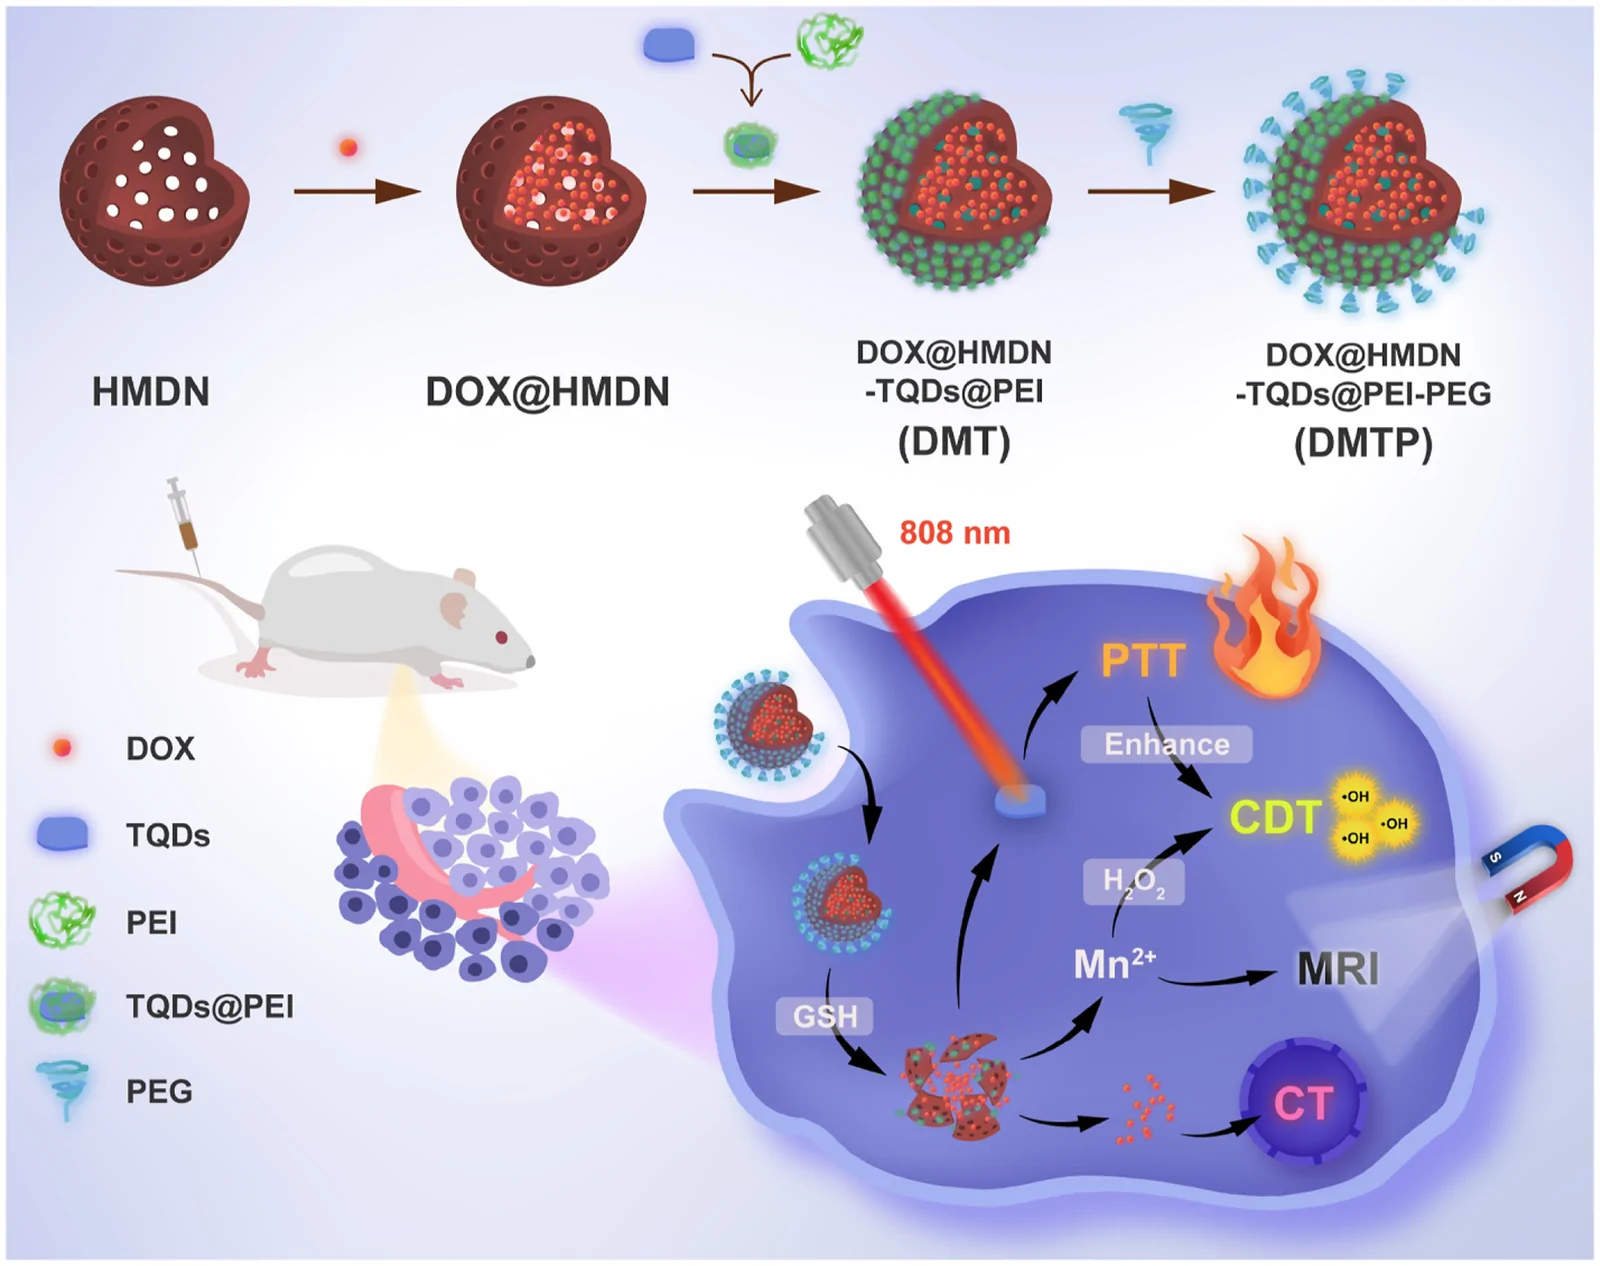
Synthesis of DOX@HMDN–TQDs@PEI–PEG (DMTP), CDT and MRI. This figure has been adapted from ref. 132 with permission from Elsevier, copyright 2024.
